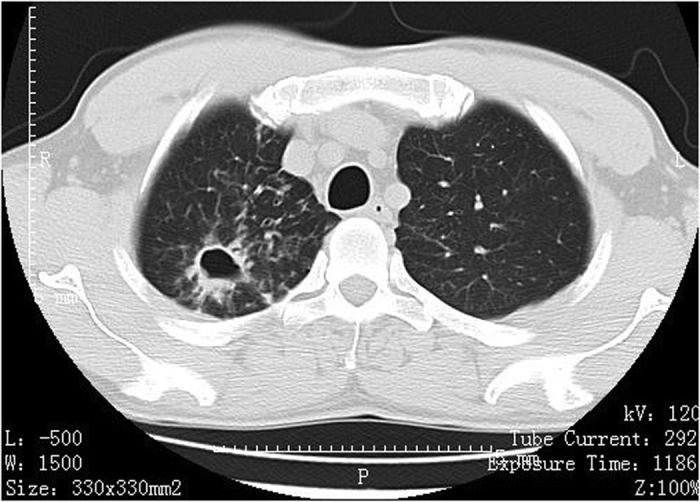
M. kansasii* lung disease in a 46-year-old man. Chest CT scan shows a cavity and centrilobular nodules in right upper lobe.
